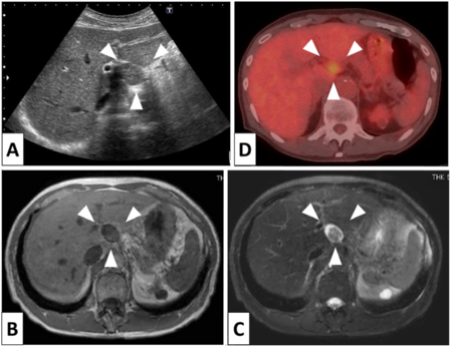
The results of Abdominal ultrasonography and weighted images of MRI. (A) Abdominal ultrasonography revealed a 2.8 × 2.5 cm solid tumor between hepatic hilus and common hepatic artery. (B) T1-weighted image of MRI showed a heterogenous low-intensity lesion. (C) T2-weighted image of MRI showed a heterogenous high-intensity lesion except for the central area. (D) PET/CT showed a low FDG-uptake mass (max SUV = 3.6).
